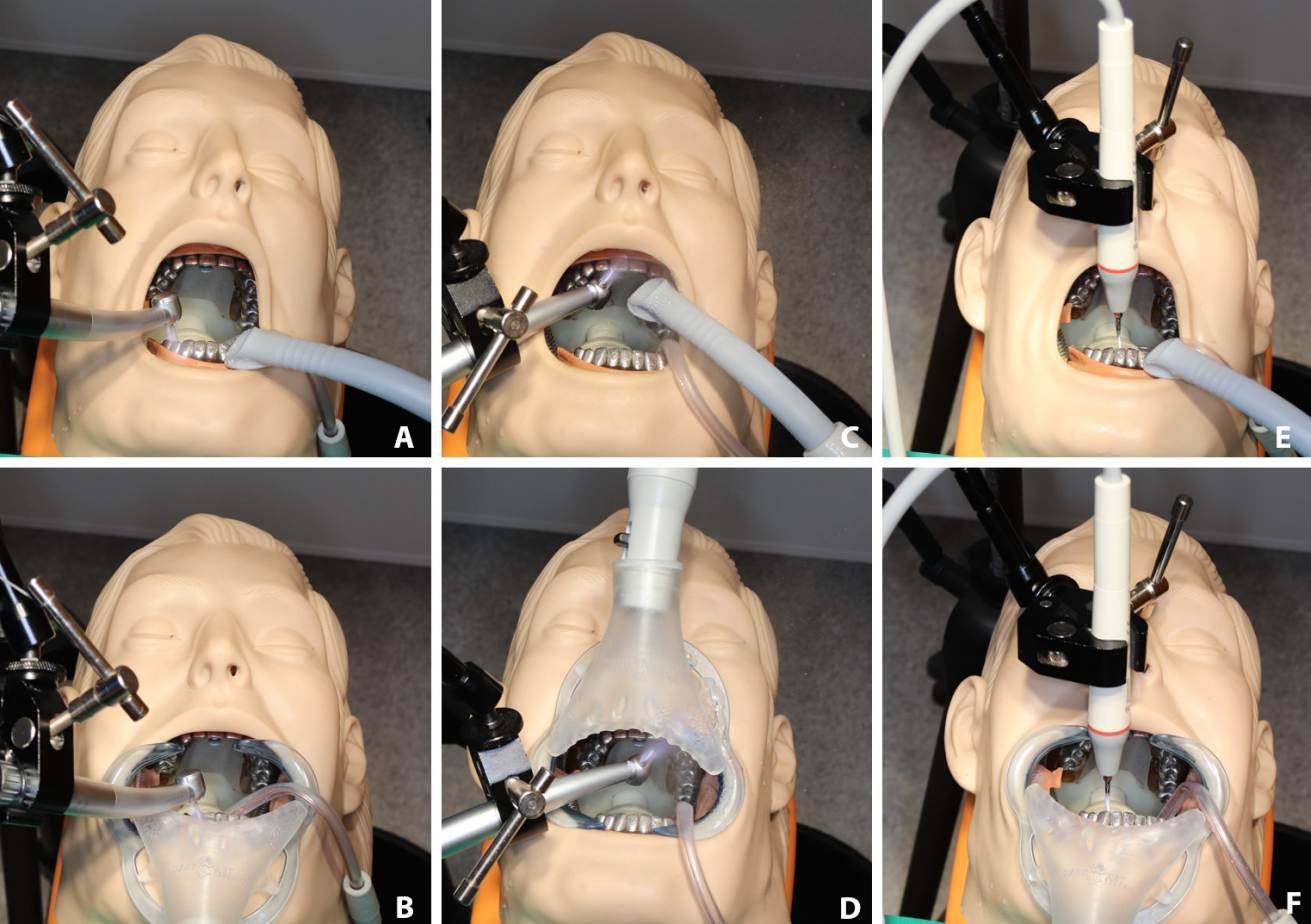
Setups for the modeling of the different clinical scenarios. A) indirect spray with high volume evacuator (IS-HVE), B) indirect spray with aerosol exhaustor (IS-AE), C) direct spray with high volume evacuator (DS-HVE), D) direct spray with aerosol exhaustor (DS-AE), E) ultrasonic scaler with high volume evacuator (US-HVE), F) ultrasonic scaler with aerosol exhaustor (US-AE).
